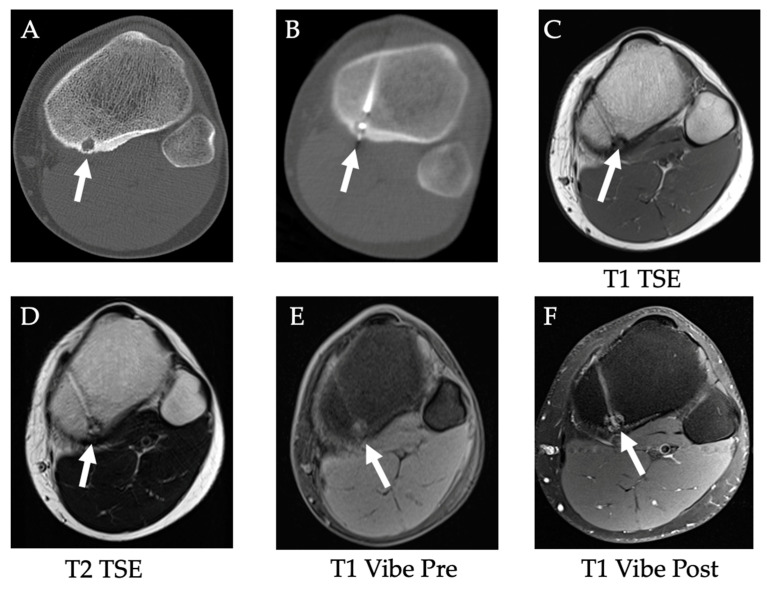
Radiofrequency ablation of osteoid osteoma. (A) A well-corticated osteoid osteoma with hypodense nidus (white arrow) in the posteromedial tibial metaphysis, (B) treated with RFA. (C–F) 5-month follow-up MR images show treated osteoid osteoma without residual lesion.
